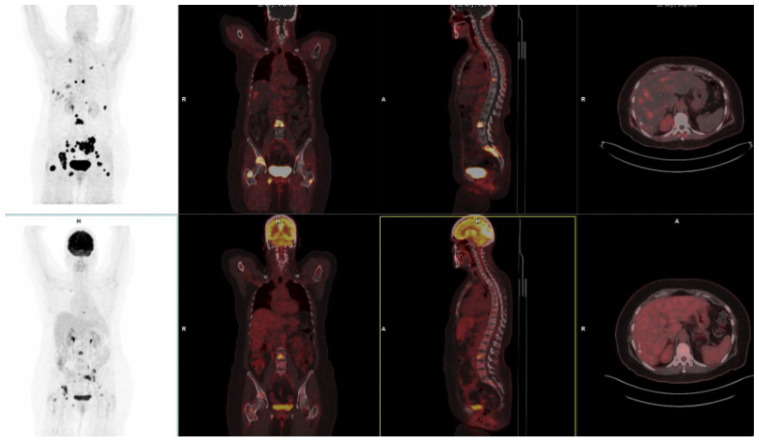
^68^Ga-FAPI-46 (top panel) and ^18^F-FDG PET/CT (bottom panel) images of a 60-year-old female with luminal A breast cancer (ER + ve, PR + ve, Ki-67 60%), demonstrating superior detection of visceral metastasis with FAPI compared to FDG. ^68^Ga-FAPI (top panel) shows multiple foci of intense tracer accumulation in the liver, multiple ribs, vertebrae, and pelvis. Corresponding ^18^F-FDG images (bottom panel) show less intense and fewer areas of tracer accumulation compared to ^68^Ga-FAPI.
